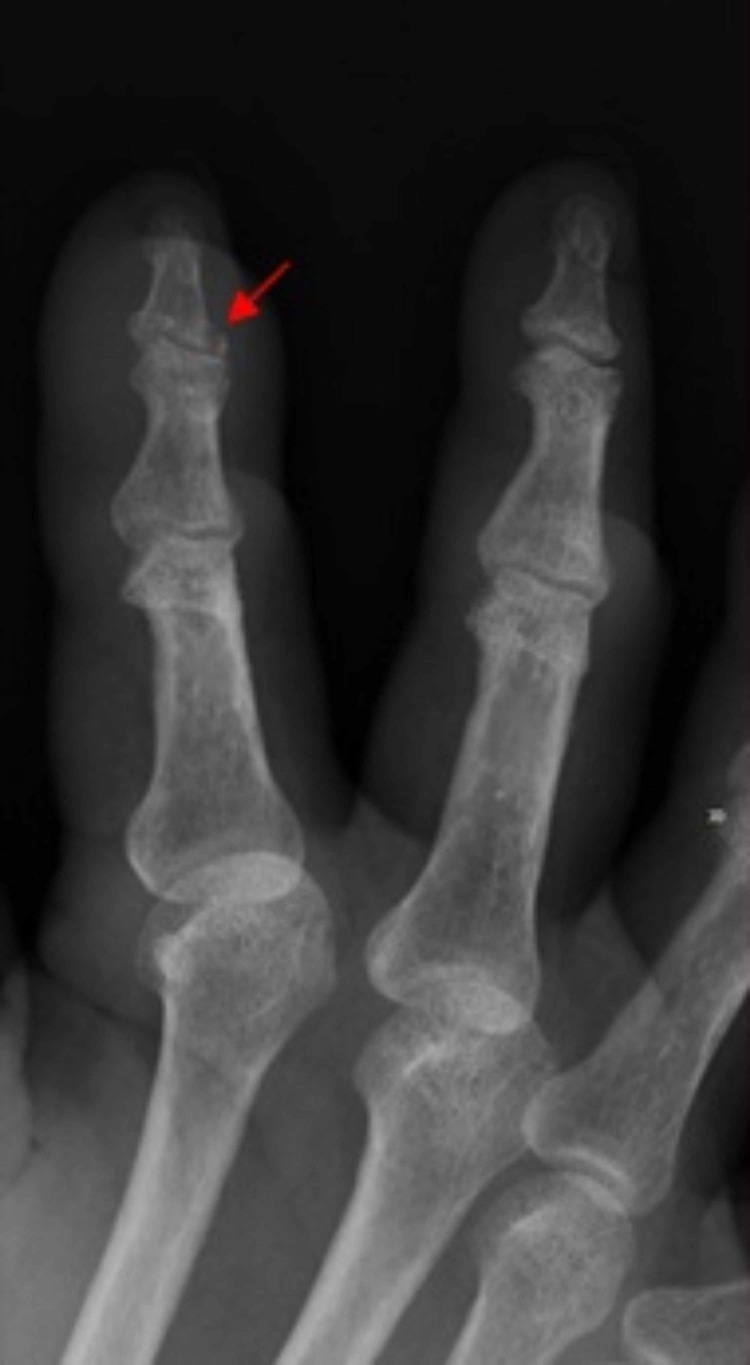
Anteroposterior radiographic imaging of the right hand, showing the distal interphalangeal joint with a classical bony erosion (red arrow).
